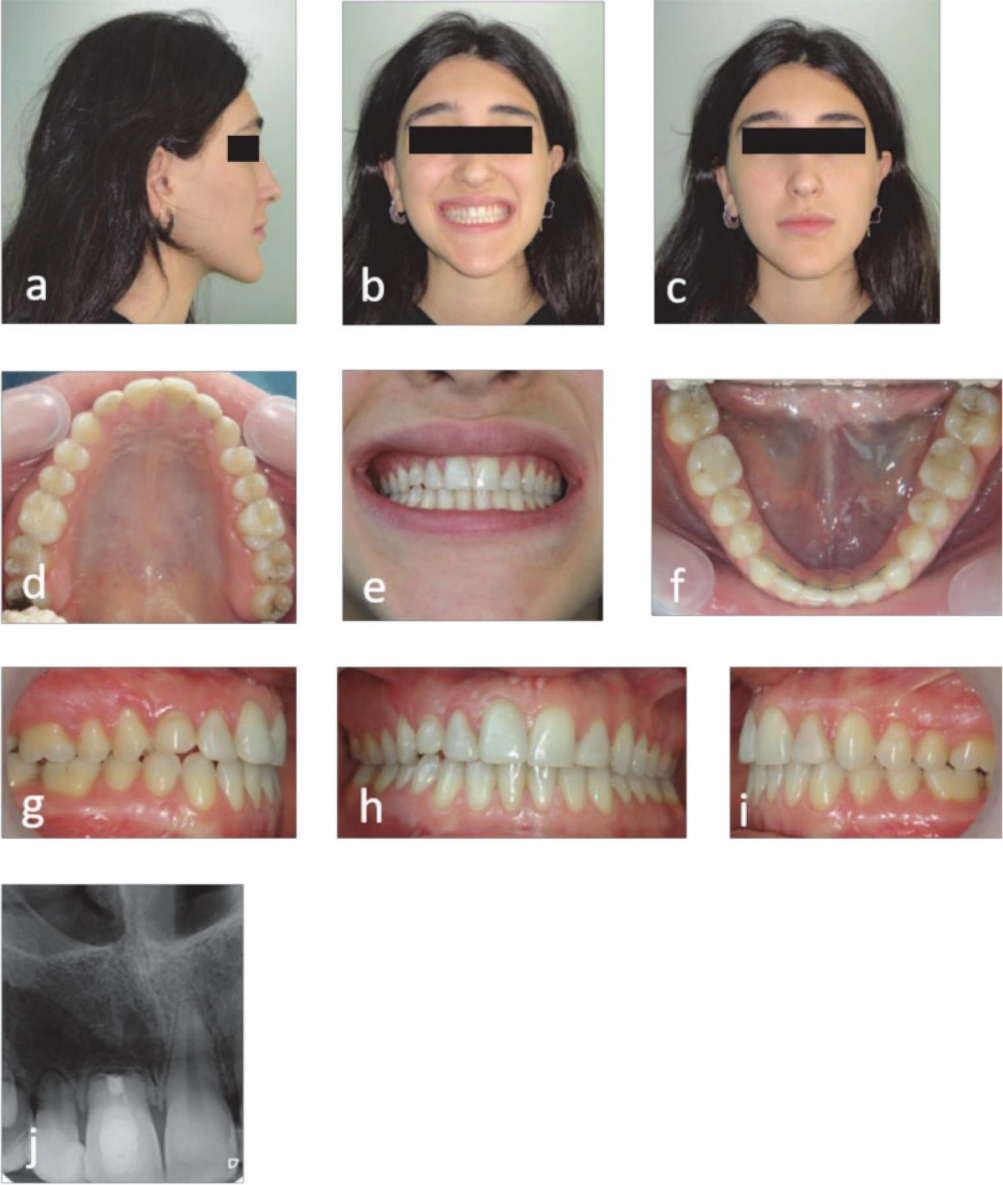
Follow‐up photographs and radiographs 10 years after endodontic microsurgery: (a–c) extraoral view with harmonious facial esthetics and smile; (d–i) intraoral view with aligned arches and Class I occlusion; and (j) periapical radiograph showing stability of the maxillary right incisor.
